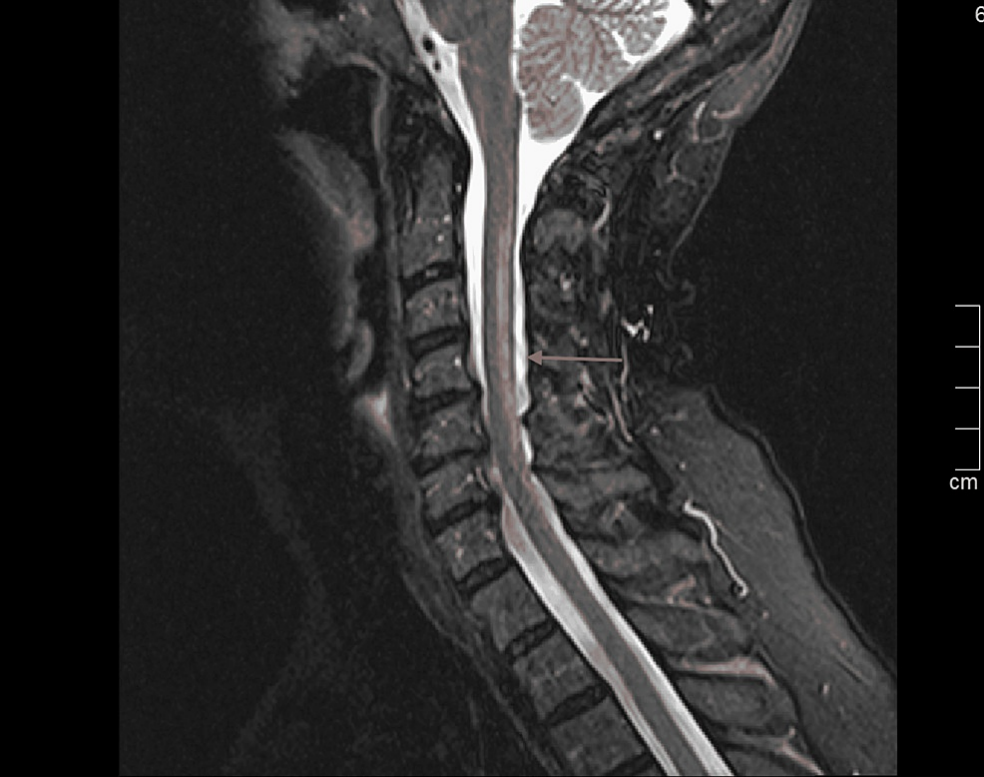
MRI cervical spine, Sagittal T2 view showing hyperintensity along with the dorsal columns at multiple levels (arrow). Some posterior disc bulges are also present.
